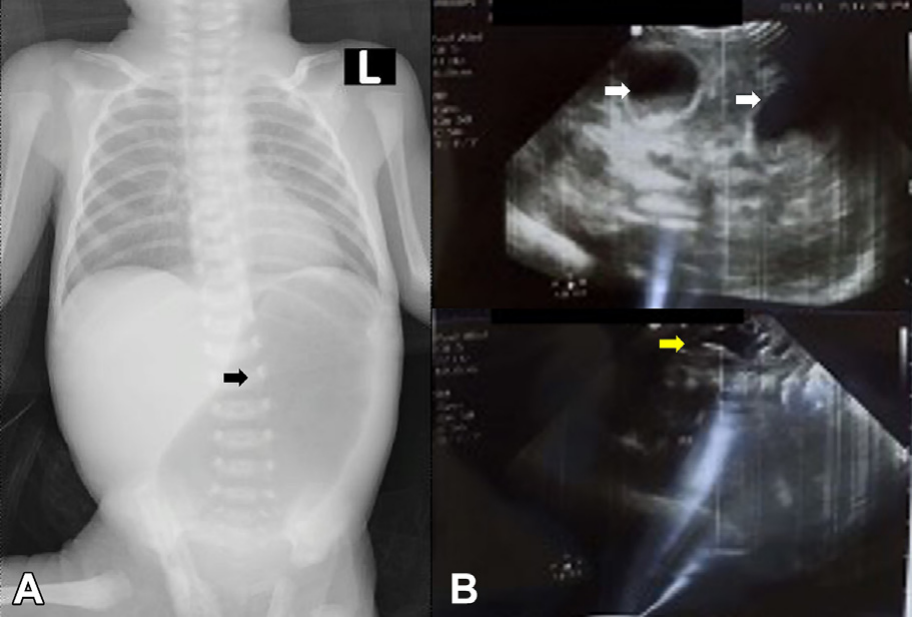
Radiological and ultrasonography examination. A, The X-ray confirmed a "single bubble appearance" suitable for pyloric atresia (*black arrow*). B, The ultrasonography examination identified bladder duplication (indicated by a *white arrow*) and left hydronephrosis characterized by the widening of the pelvicalyceal system and thinning of the calyx (indicated by the *yellow arrow*).
